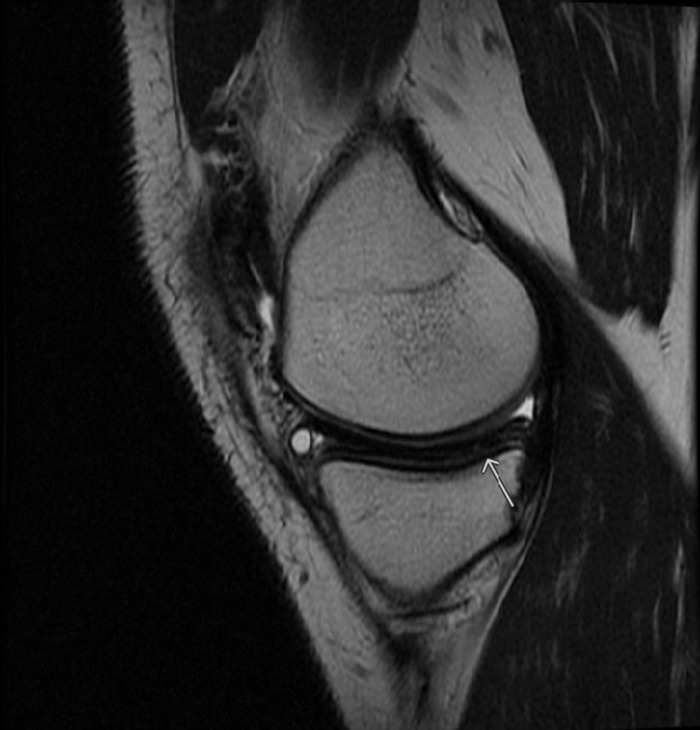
Sagittal T2 magnetic resonance image shows a horizontal oblique undersurface tear of the posterior horn of the medial meniscus (arrow).
